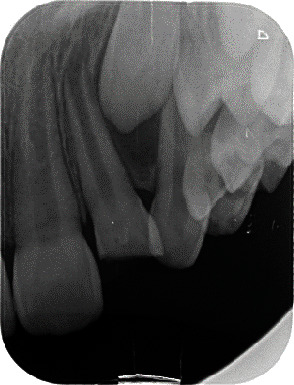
Periapical radiograph of the fractured tooth (Number 22).
